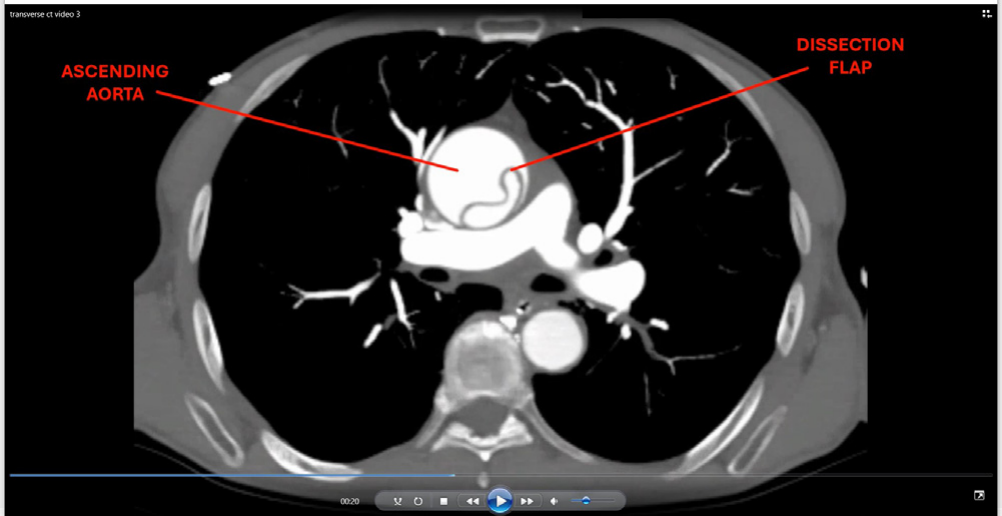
Transverse-axis computed tomography angiography showing a dissection flap in the ascending aorta with extension into brachiocephalic, left common carotid, and left subclavian arteries.
